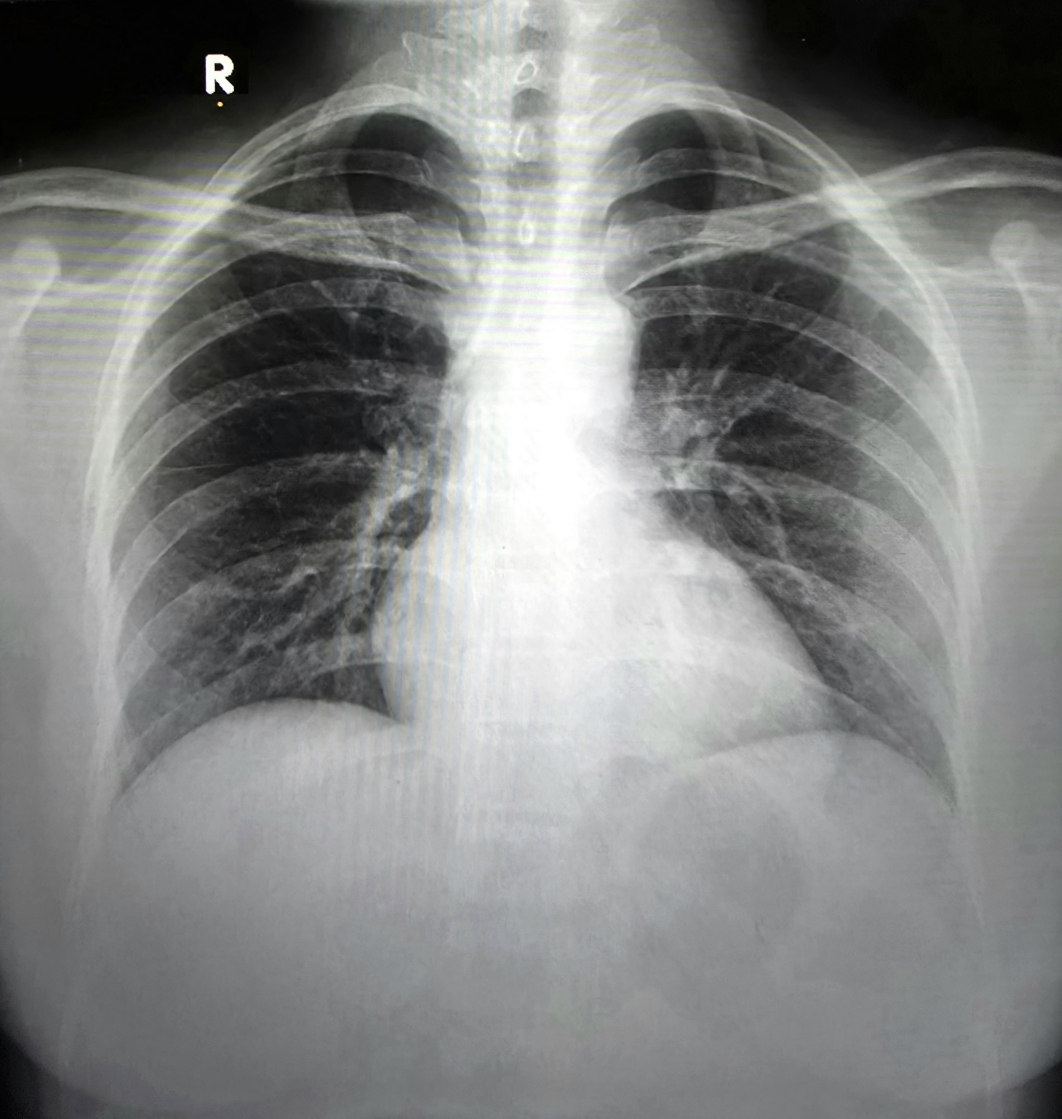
Chest X-ray postero-anterior view
